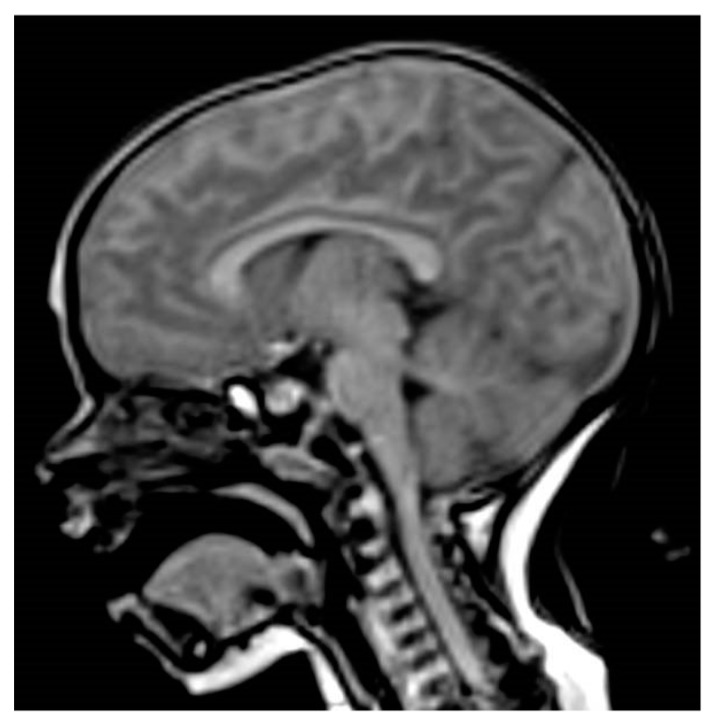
Sagittal pituitary MRI image showing hyper-intense enlarged anterior pituitary (T1-weighted sequence).
